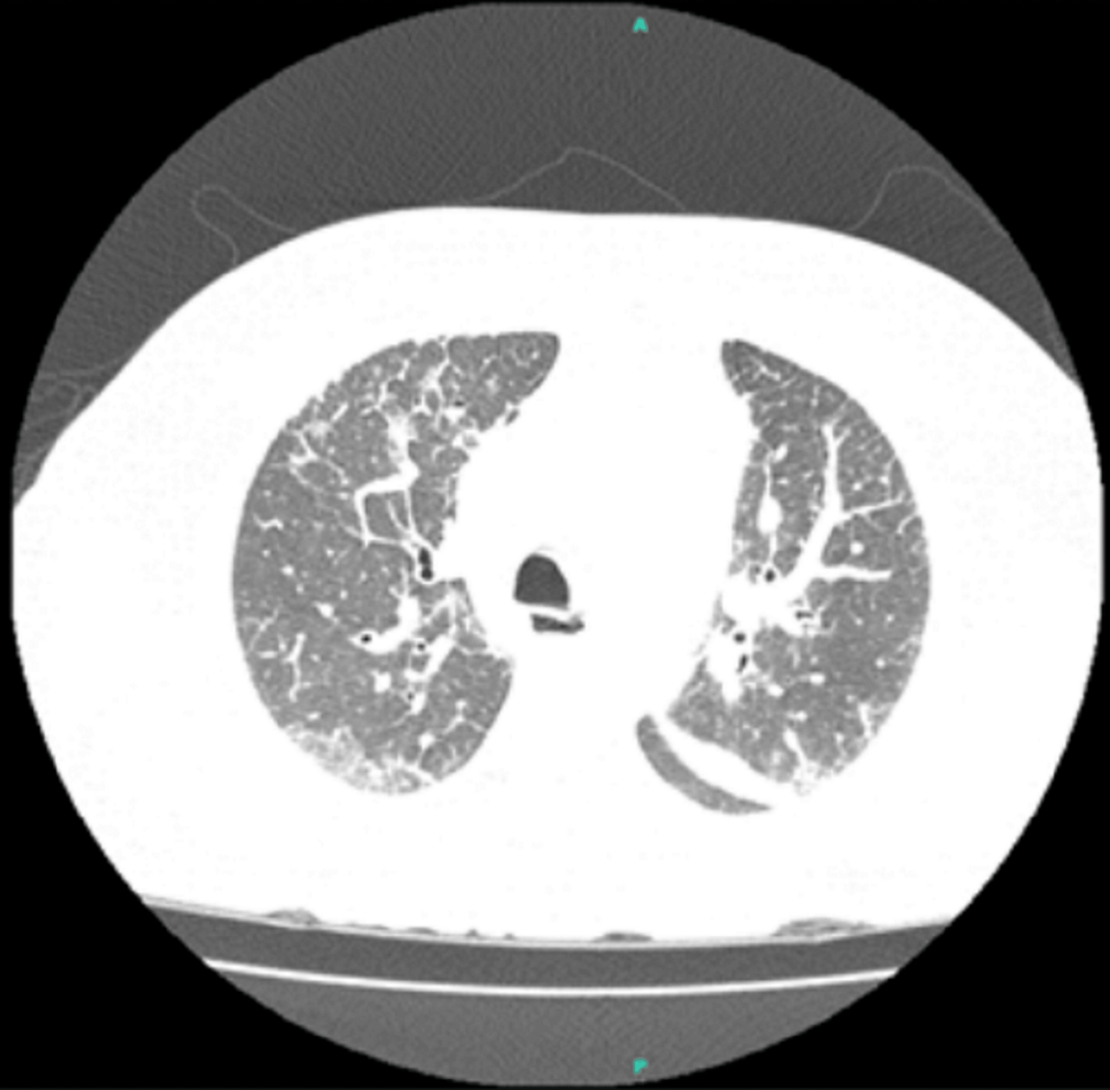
Computed tomography of the lung. The findings were consistent with interstitial lung disease (ILD), including areas of ground-glass opacities and reticular markings, as well as evidence of pulmonary edema characterized by septal thickening and alveolar opacities.
